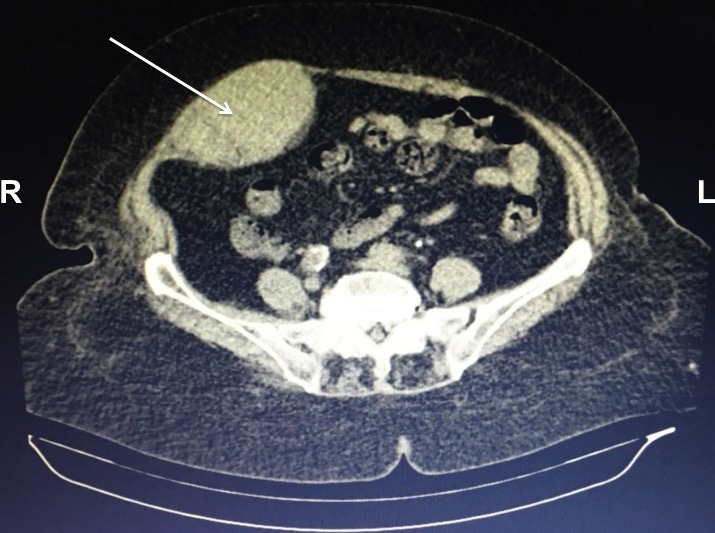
Computed tomography of abdomen showing hematoma (arrow) within rectus sheath; L: left; R: right
